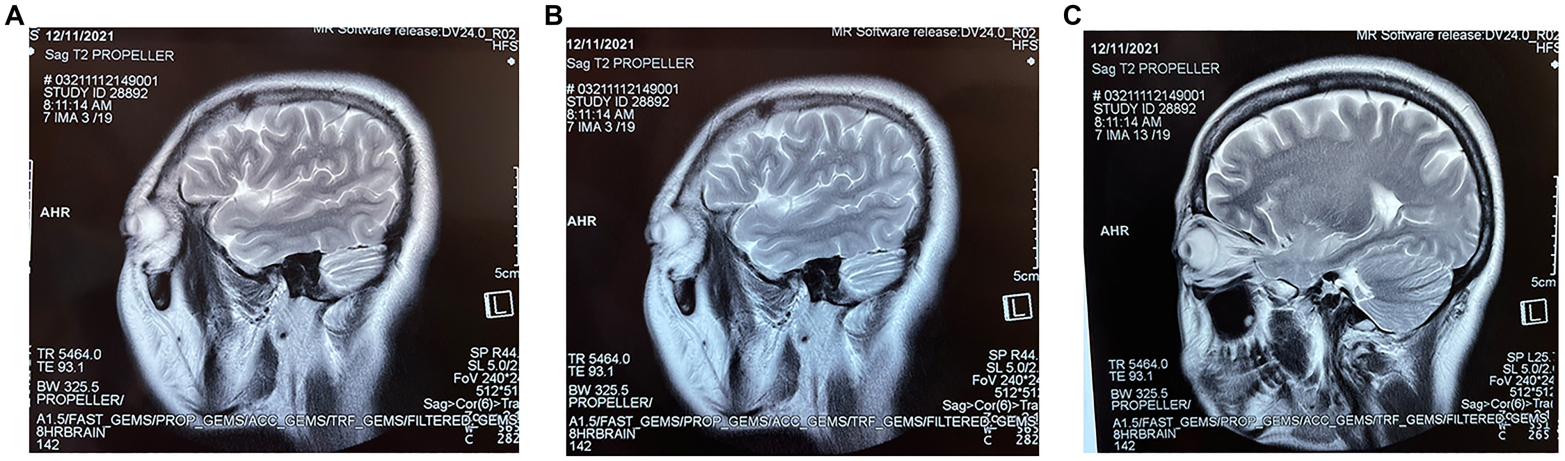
(A–C) are three different sections of the sagittal position of the first MRI at the patient’s admission.
